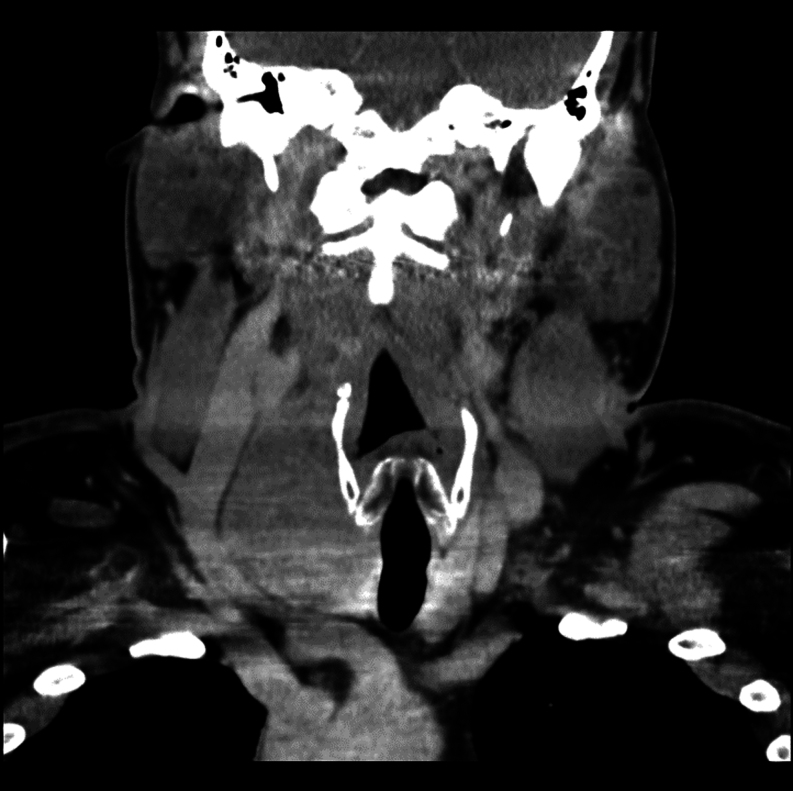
CT of the neck, performed after the administration of intravenous contrast material, revealed a 7.7 × 4.3 × 4.4 cm (cephalocaudal by transverse by AP dimensions) right thyroid mass with slight leftward displacement of the larynx and subglottic trachea.
